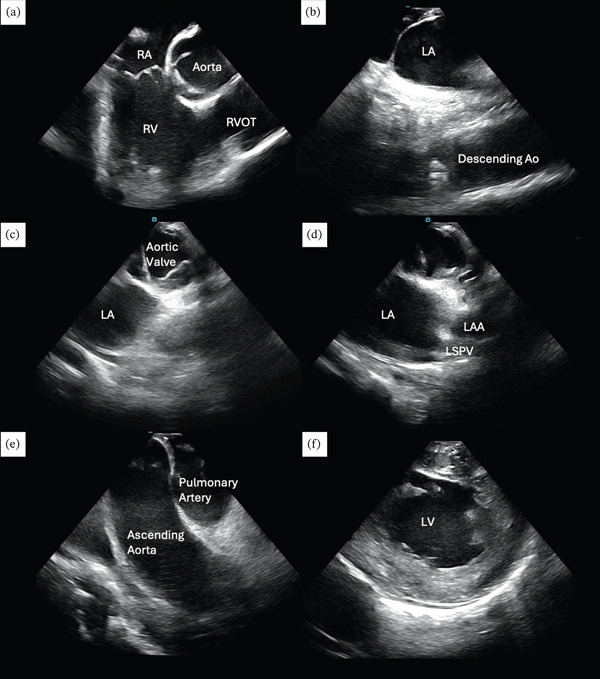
Intracardiac echocardiography of a patient with dextrocardia. (a) Home view. (b) Interatrial septum and descending aorta. (c) Aortic valve in short‐axis view. (d) Left atrial appendage and left superior pulmonary vein. (e) Ascending aorta and pulmonary artery. (f) Left ventricle. Abbreviations: LA, left atrium; LAA, left atrial appendage; LSPV, left superior pulmonary vein; LV, left ventricle; RA, right atrium; RV, right ventricle; RVOT, right ventricular outflow tract.
